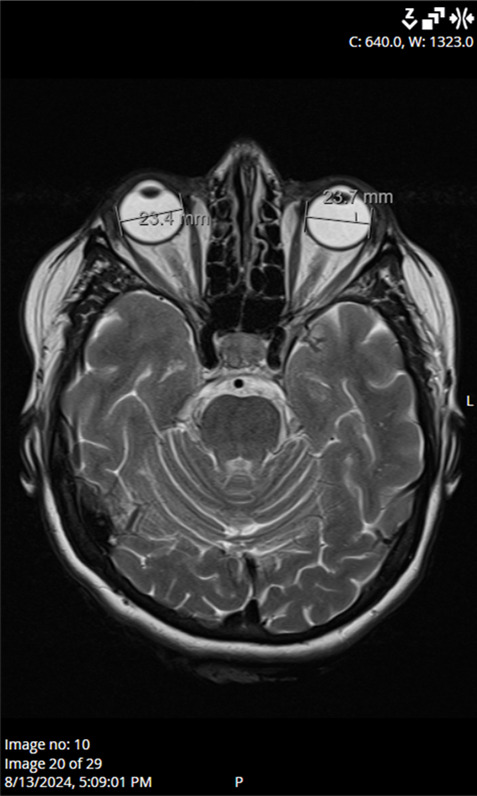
MRI scan of the brain and orbits shows no pathological findings, effectively ruling out an anatomical cause for the optic disc edema.
